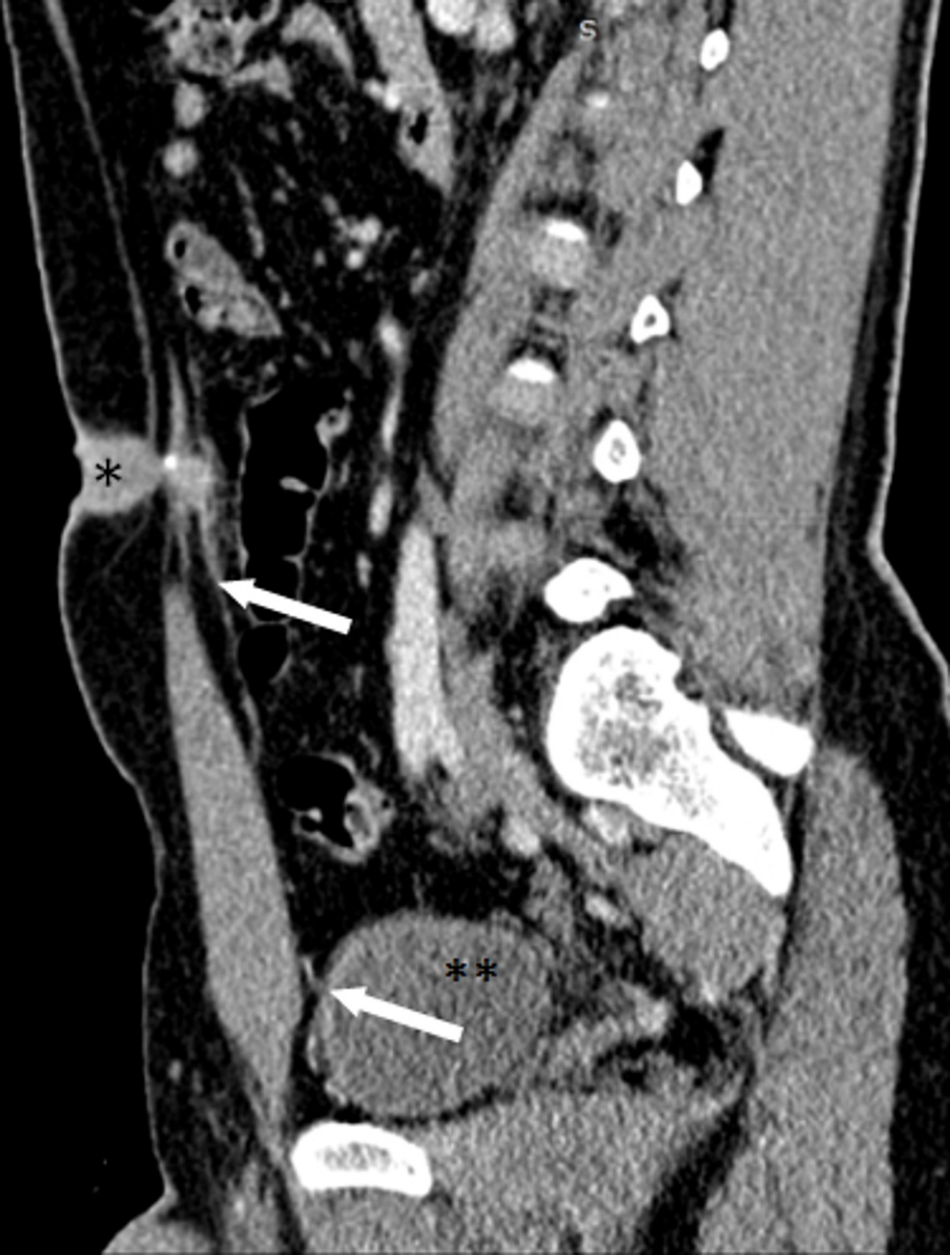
Sagittal reconstruction of a contrast-enhanced abdominal computed tomography showing the tubular collection (*) with a peripheral calcification extending from the umbilicus to the pre-peritoneal space. A linear band (White arrows) connecting the collection’s postero-inferior end to the bladder () and corresponding to the medial umbilical ligament is noted.
